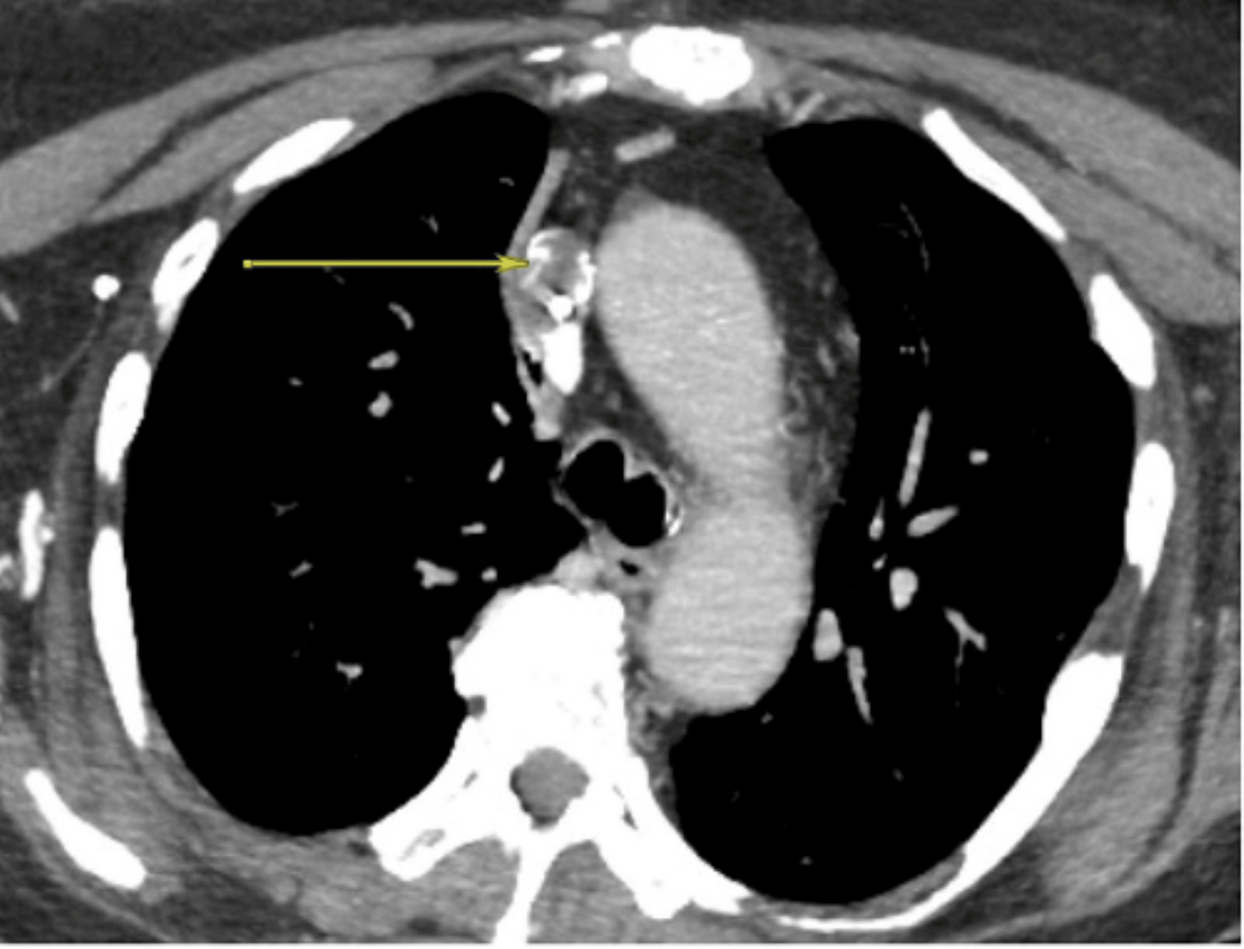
CTA thorax with IV contrast (transverse view) demonstrating a thrombus (yellow arrow) in the SVC, resulting in irregular filling defects. CTA, computed tomography angiography; IV, intravenous; SVC, superior vena cava.
